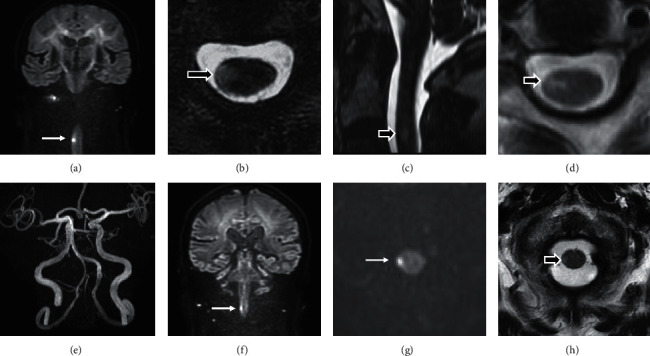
Magnetic resonance imaging (MRI) of spinal infarctions in two cases. The lesion for case 1 is presented in (a–e). (a) Thin-section coronal diffusion-weighted imaging (DWI), showing a high-intensity lesion of the cervical spine (arrow). (b and c) 3D fast imaging employing steady-state acquisition; MRI showing an upper cervical lesion. Axial (b) and sagittal (c); MRI showing a small, high-intensity lesion (arrows) at the level of the C2/3 intervertebral disc. (d) Axial, T2-weighted image of the cervical cord, examined 5 weeks after onset. (e) MR angiography of case 1, showing no apparent dissection of vertebral arteries. The MRI findings of case 2 are presented in (f–h). (f) Thin-section, coronal DWI and (g) axial DWI, demonstrating a high-intensity lesion (arrows) in the upper cervical cord. (h) T2-weighted axial image, showing a high-intensity lesion (arrow) in the right lateral funiculus at the level of the C1 vertebra.
